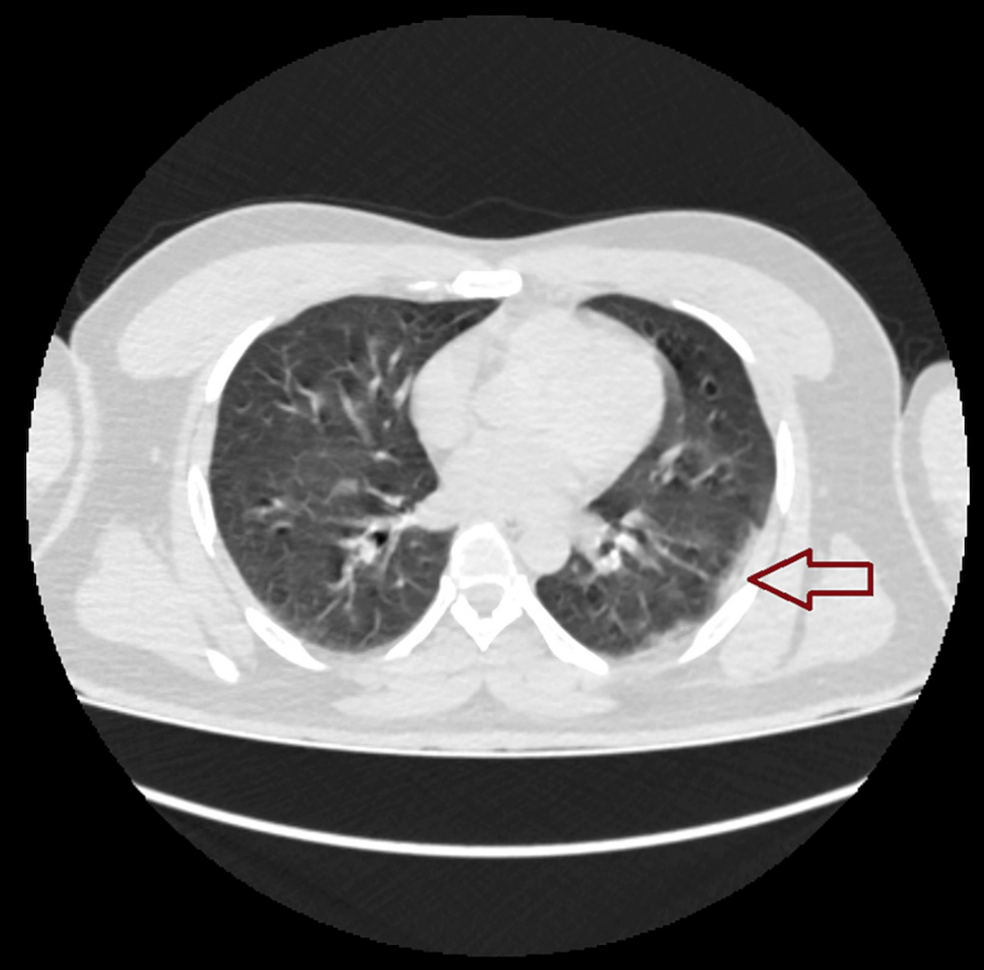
CT chest image showing ground glass opacity
